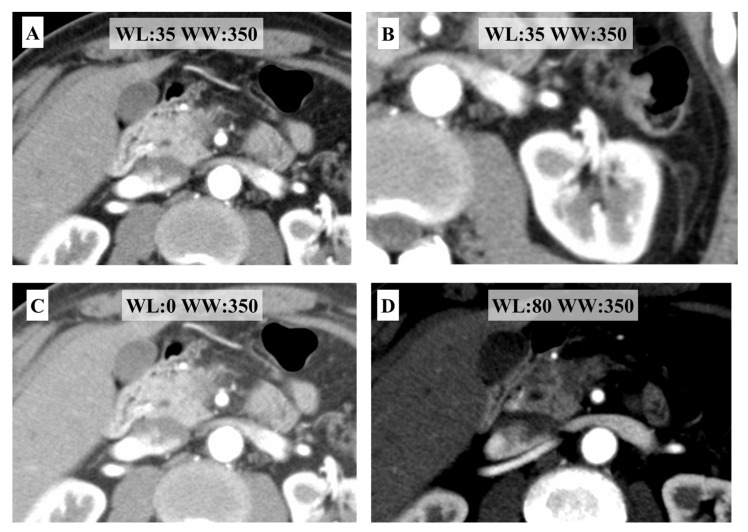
CT window settings for evaluation of extrapancreatic invasion. (A) WL 35/WW 350 (arterial phase). (B) Visualization of the Gerota fascia at WL 35/WW 350. (C) WL 0/WW 350 (overestimation). (D) WL 80/WW 350 (underestimation).
